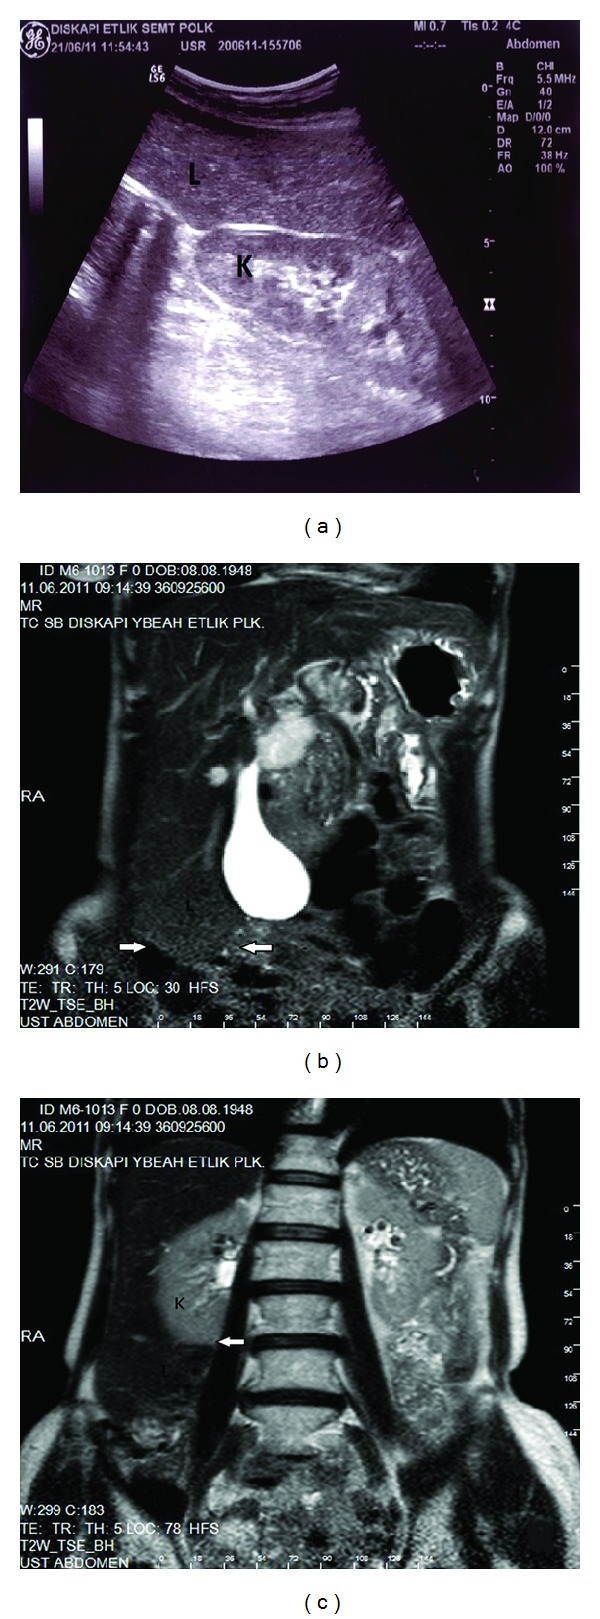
(a) There was hepatomegaly on US imaging. (b) Coronal plane of MRI showed that the inferior pole of the liver was located in the pelvic space (arrows) and (c) the inferior pole of the right kidney was covered by the liver (arrow), L: liver and K: kidney.
